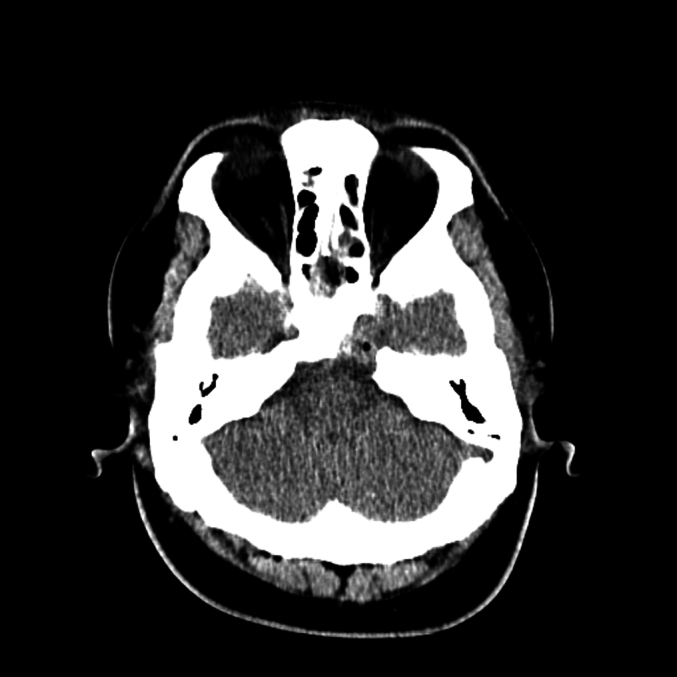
Post-operative axial CT scan confirming the absence of any remaining mass in the hypothalamic region.
